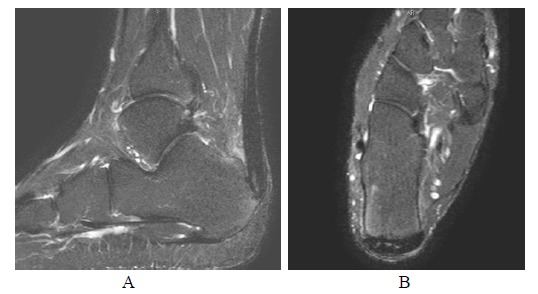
Achilles tendinosis (insertional). Abnormal T2-weighted hyperintense signal is present within the tendon near its insertion.
